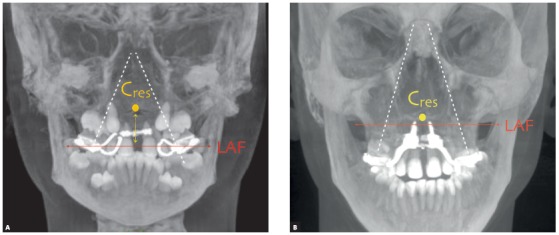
Thick CBCT coronal slice (160 mm). A) Patient after RPE; red arrow indicates line of action of force (LAF) at the level of anchorage teeth, at a certain distance from the center of resistance (Cres), in yellow. White dashed line indicates opening of maxillary bones. B) Patient undergoing MARPE: red arrow indicates line of action of force; center of resistance in yellow. Force is applied at a point very close to center of resistance of midpalatal suture. Angle of opening of maxillary bones (white dashed line) is less accentuated.
